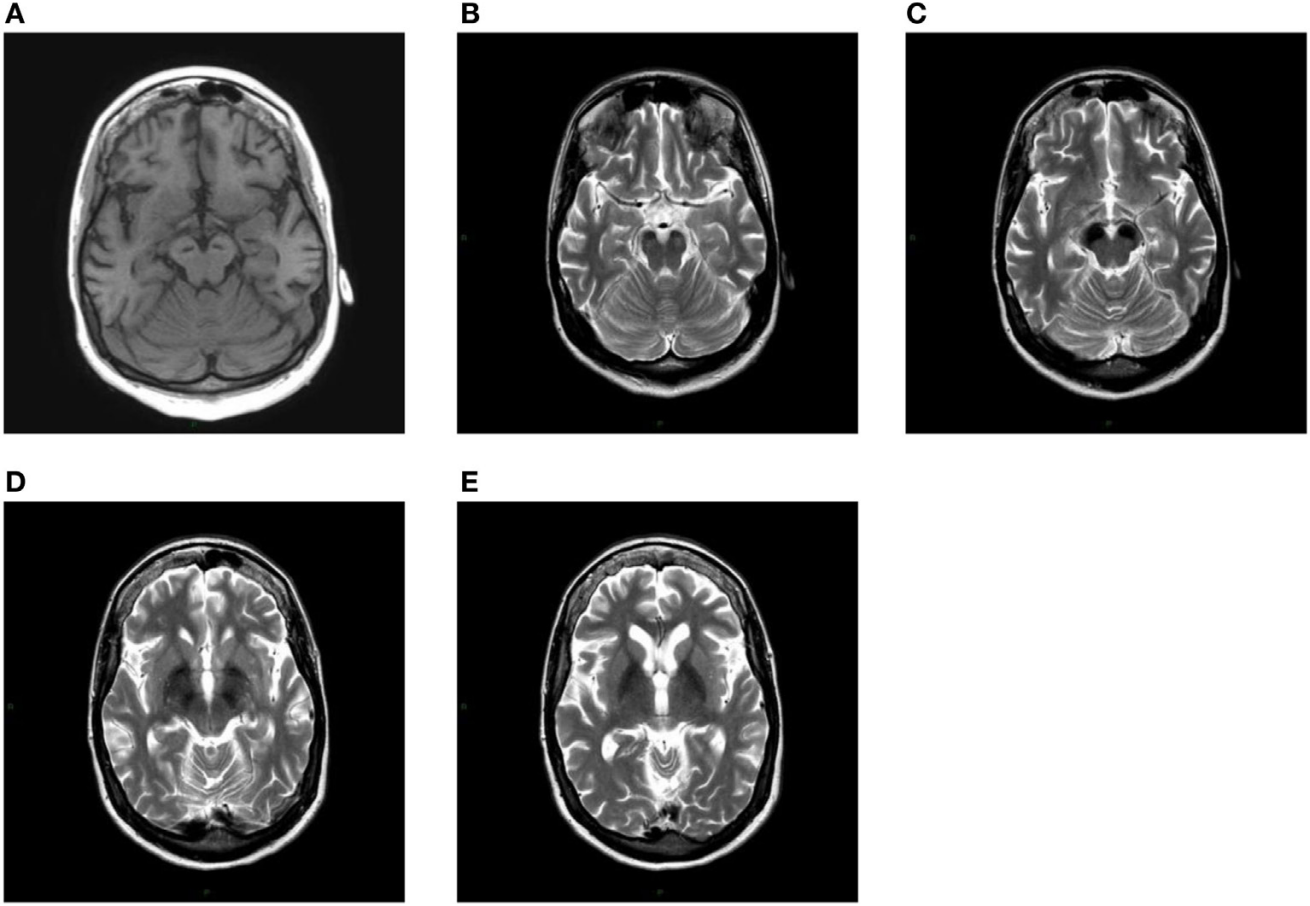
(A) T1-weighted axial image shows linear hypointensity at the level of cerebral peduncles in the substantia nigra within a mild area of hyperintensity. At the same level in the images (B,C), both T2-weighted, a striking hypointensity can be seen in both substantiae nigrae. A more cranial axial T2-weighted section shows a marked bilateral hypointensity of the globus pallidus (D,E).
